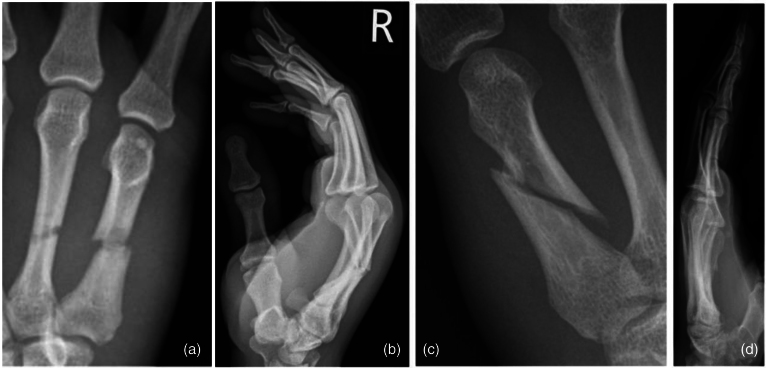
Sample of radiographs included in the survey to assess preferred treatments: (a) anteroposterior view transverse metacarpal shaft fractures (MSF) ring finger, (b) lateral view transverse MSF ring finger, (c) anteroposterior view long oblique MSF little finger and (d) lateral view long oblique MSF little finger.
